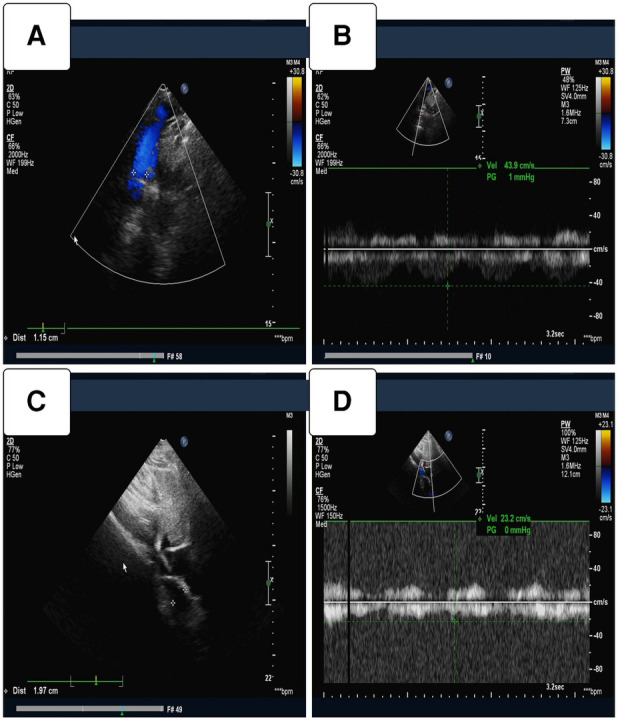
Transthoracic echocardiography in April 2026 (26 years post-TCPC). (A) Color Doppler imaging of the SVC-RPA anastomosis demonstrating patency with a diameter of approximately 12 mm. (B) Pulsed-wave Doppler of the SVC-RPA anastomosis showing unobstructed flow velocity of 43.9 cm/s. (C) Two-dimensional grayscale imaging of the IVC conduit showing a diameter of approximately 19 mm. (D) Pulsed-wave Doppler of the IVC conduit showing unobstructed flow velocity of 23.2 cm/s.
